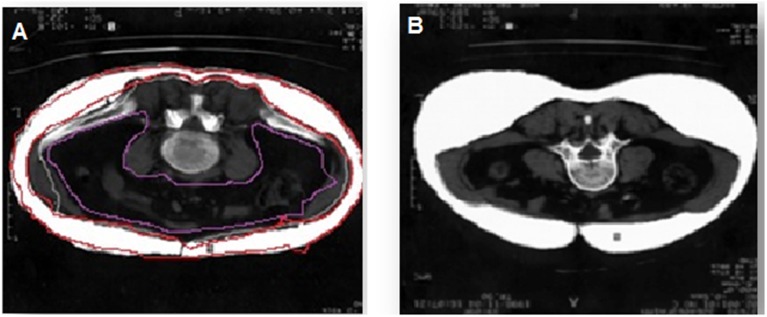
Diagnosis of visceral obesity via multi-spiral computed tomography. (A) Patient with obesity, (B) patient without obesity.
